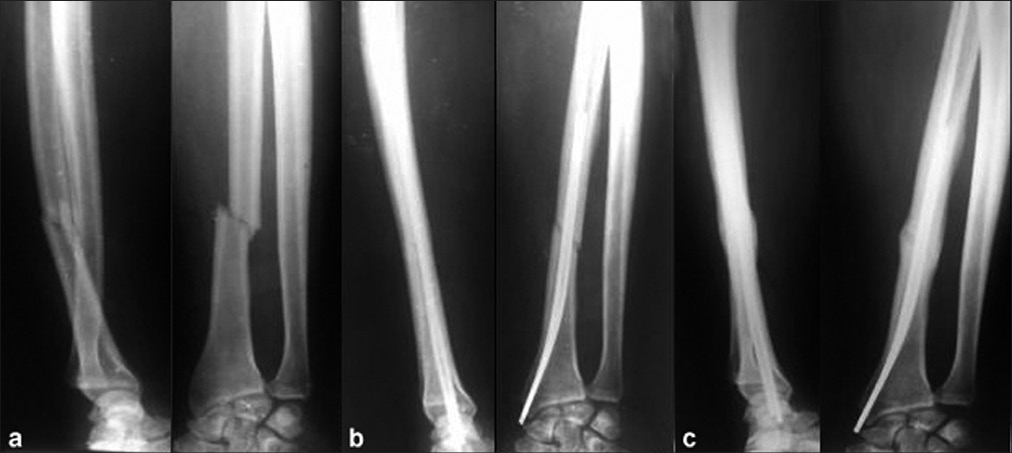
Lateral and anteroposterior X-ray of right forearm showing (a) a Galeazzi fracture dislocation treated with closed elastic stacked intramedullary nailing (b) early union and good alignment at 12 weeks followup, (c) progressing to sound fracture union and well aligned DRUJ at 12 months follow-up
